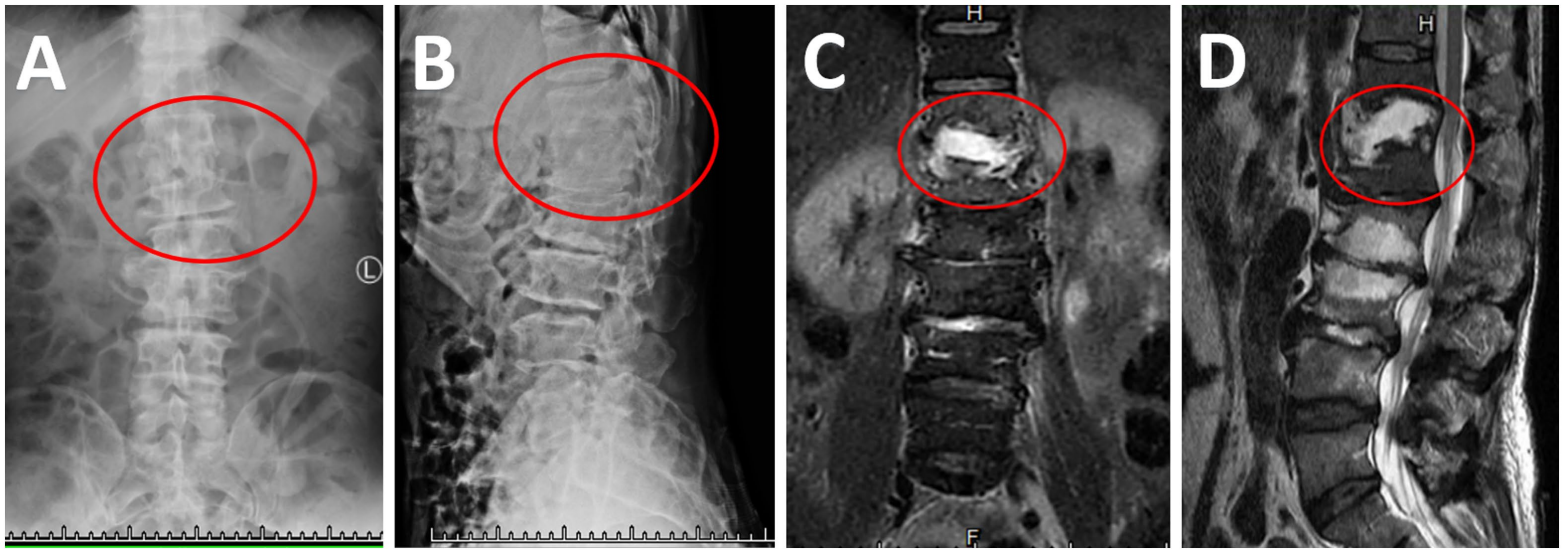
Imaging evidence of T12/L1 infection. (A,B) Indicate osteolysis of the T12 and L1 vertebral bodies on X-ray. (C,D) Show abnormal signal intensity in the T12 and L1 vertebral bodies and intervertebral disc on MRI, indicating pyogenic infection.
